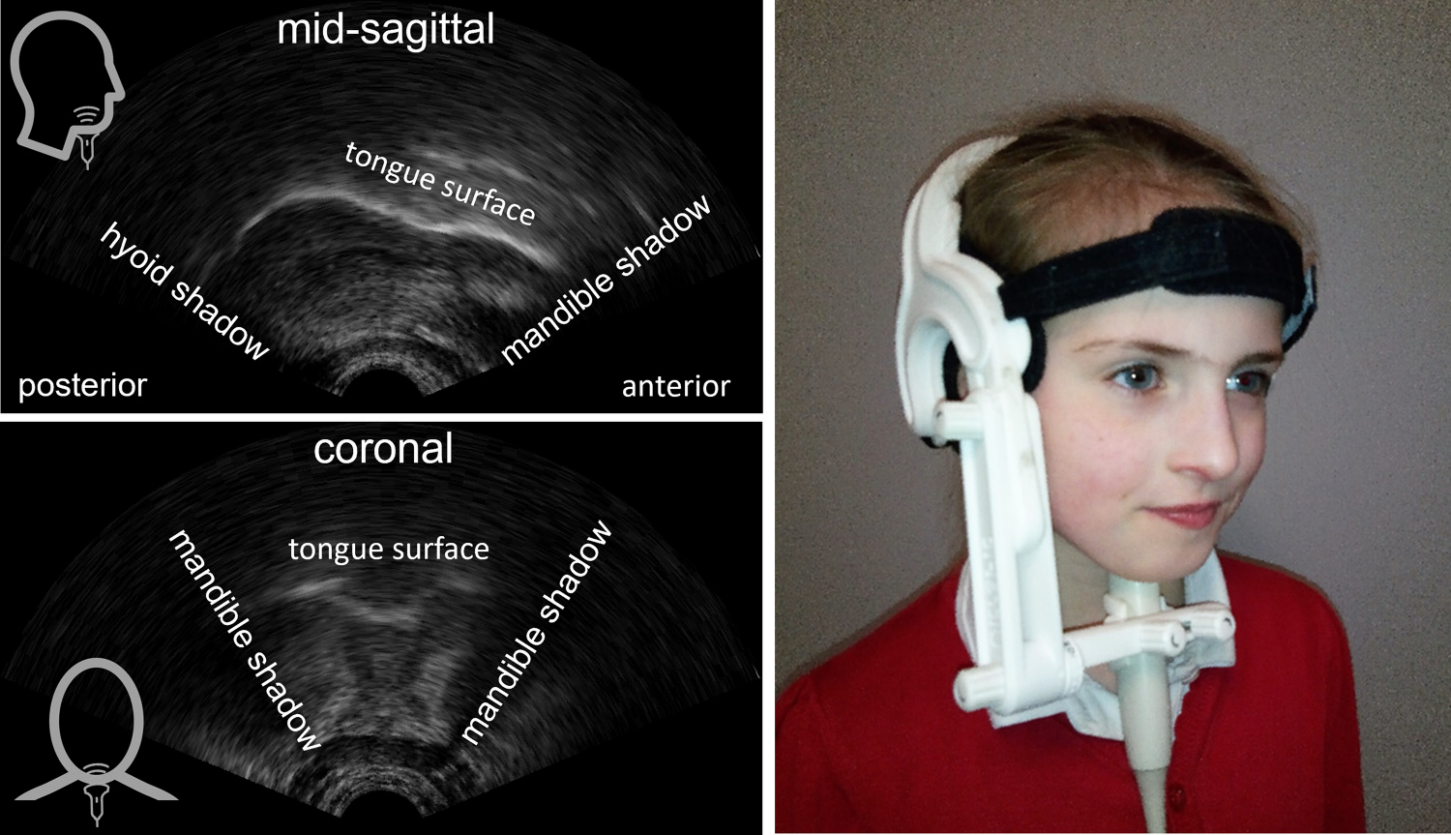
Ultrasound images of the tongue. Top left: mid-sagittal image with the tongue tip to the right. Bottom left: coronal image. In both images the brightest white line is the tongue surface. Right: Ultrafit headset with probe positioned for mid-sagittal view.
